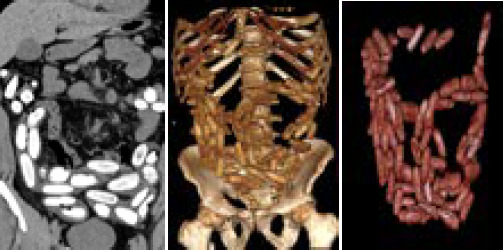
A. CT abdomen-coronal image, B. 3D volume rendering image, C. 3D volume rendering image with bone subtraction.
